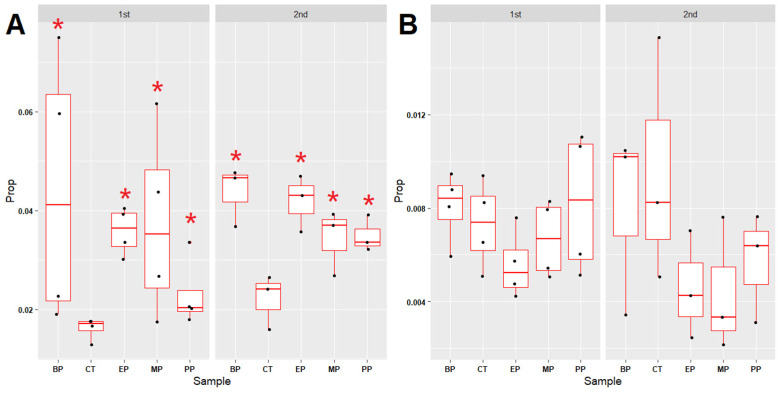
Proportion changes of microbial communities in the paraben-treated river sediments. (A) Bacterial genera associated with xenobiotics degradation. (B) Microbial genera with potential pathogenic bacteria. “1st” indicates the meaning of the period between week 0 and week 8. “2nd” indicates the meaning of the period between week 8 and week 17. Red star indicates the *p* value of the Mann–Whitney U test < 0.05 (compared with control (CT)). Prop: proportions of microbial genera. “M00xxx” indicates the meaning of the KEGG module ID number. MP: methylparaben, EP: ethylparaben, PP: propylparaben, BP: butylparaben, CT: control.
